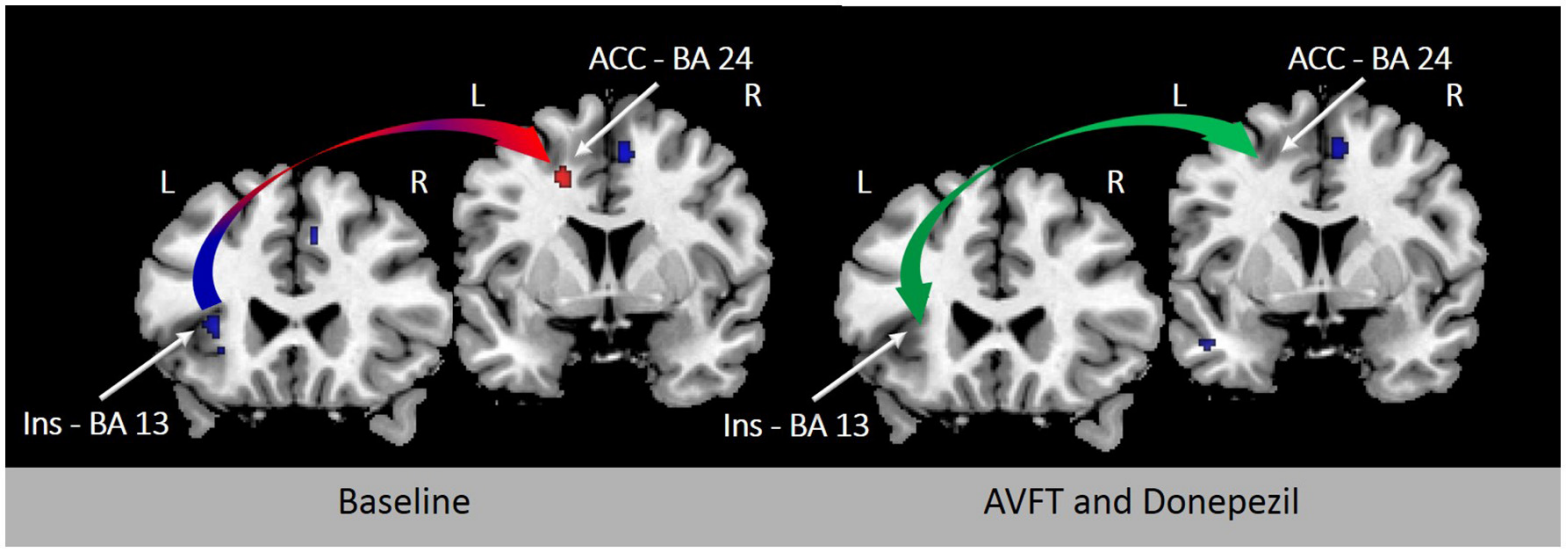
Resting [^18^F]-fluorodeoxyglucose positron emission tomography (^18^FDG-PET) before and after treatment in a 44-year-old woman with chronic post-stroke foreign accent syndrome (FAS). Rates of metabolic activity in this single patient were compared with 18 healthy control subjects (female/male: 11/7; mean age ± SD: 56.6 ± 5.7 years; age range: 47–60 years). The left panel shows T1-weighted coronal sections depicting significant hypometabolism in the left deep frontal operculum and dorsal anterior insula (Talairach and Tournoux peak coordinates: *x* − 36 *y* 14 *z* 16) (blue arrow) with significantly increased compensatory metabolic activity in the left ACC (Talairach and Tournoux peak coordinates: *x* −18 *y* 8 *z* 40) (red arrow) before treatment (Moreno-Torres et al., 2013). Clusters in baseline ^18^FDG-PET were significantly larger with family-wise error correction (*p* < 0.05) in comparison to healthy controls. Deficient phonetic error awareness and monitoring responsible from FAS in this patient were treated with audiovisual feedback training (visually-guided using Praat—(Boersma and Weenink, 2010)—and adjusting patient's emissions to F0 contours of sentence-models) alone and in combination with a cholinergic drug (donepezil, 5 mg/day). An almost complete return of accent to its premorbid characteristics was observed after combined treatment and these changes correlated with normalization of metabolic activity in the left AIC and ACC (green arrow; right panel; Berthier et al., 2009). BA indicates Brodmann area.
